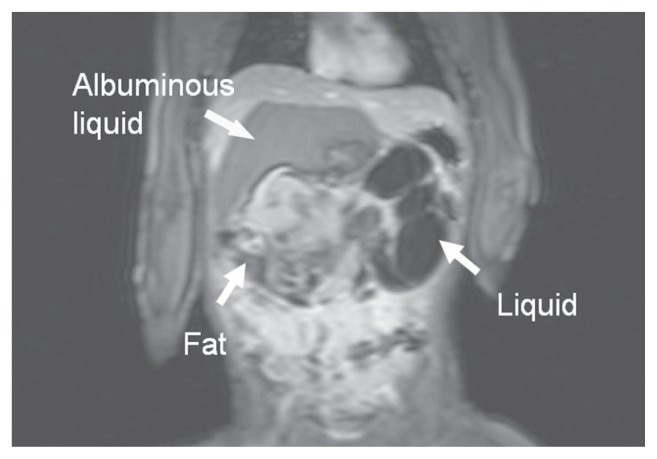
Solid—cystic teratoma containing different types of tissue like fat, albuminous liquid and other solid parts (MRI, T1-gradient echo sequence, coronal).
